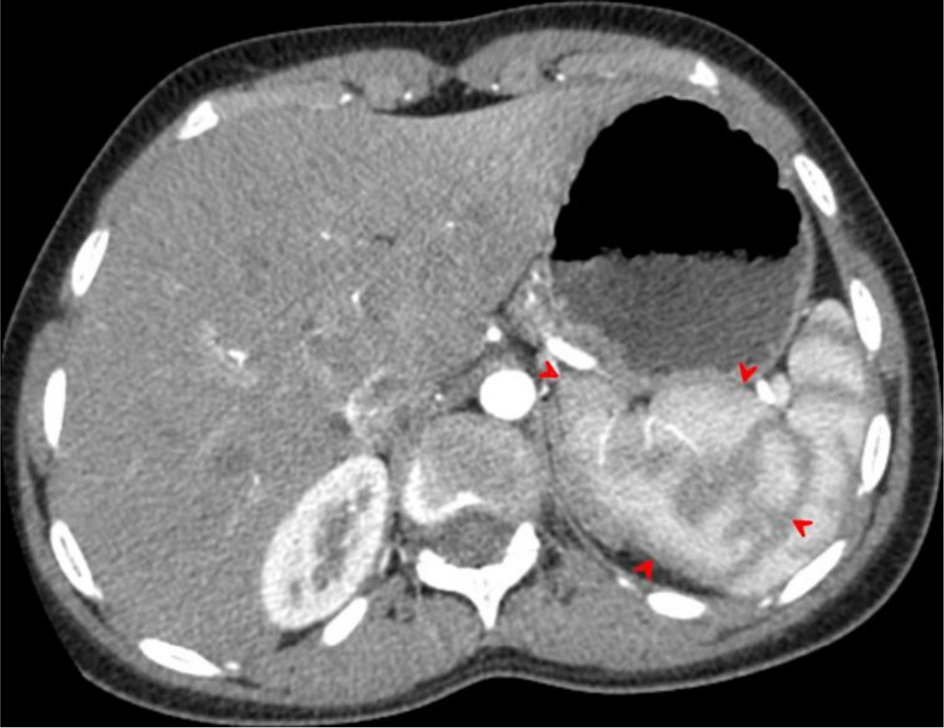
Axial contrast-enhanced CT image acquired during the arterial phase through the upper spleen demonstrates the medial internal lobe of the spleen (red arrowheads) shows the characteristic mottled appearance, extending medially displacing the upper pole of left kidney downwards and gently indenting the posterior aspect of the gastric fundus. The upper pole of the right kidney seen while the upper pole of the left kidney not demonstrated (displaced downwards).
